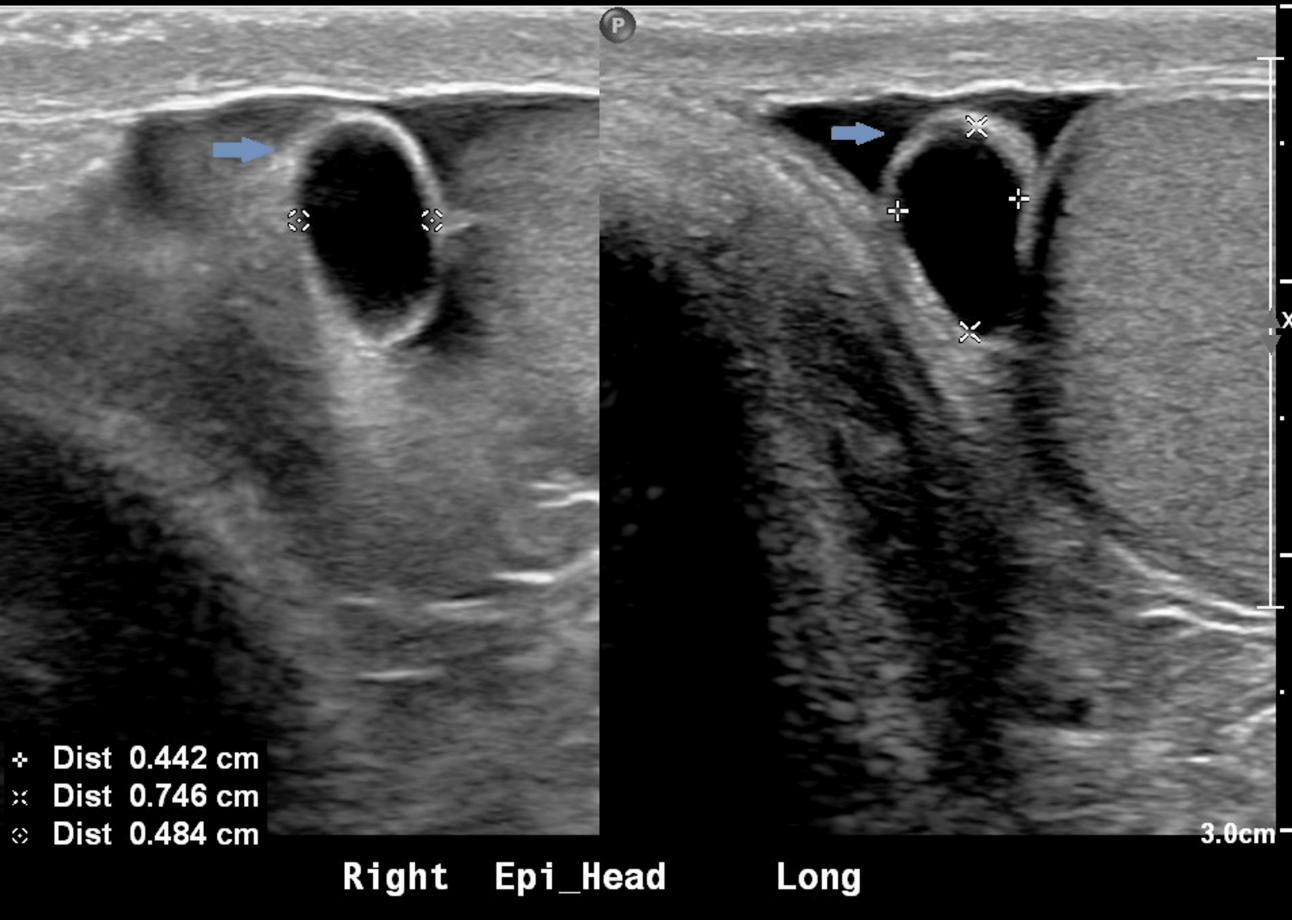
The arrow shows an 8mm right epididymal head cyst but otherwise unremarkable testicular ultrasound.
